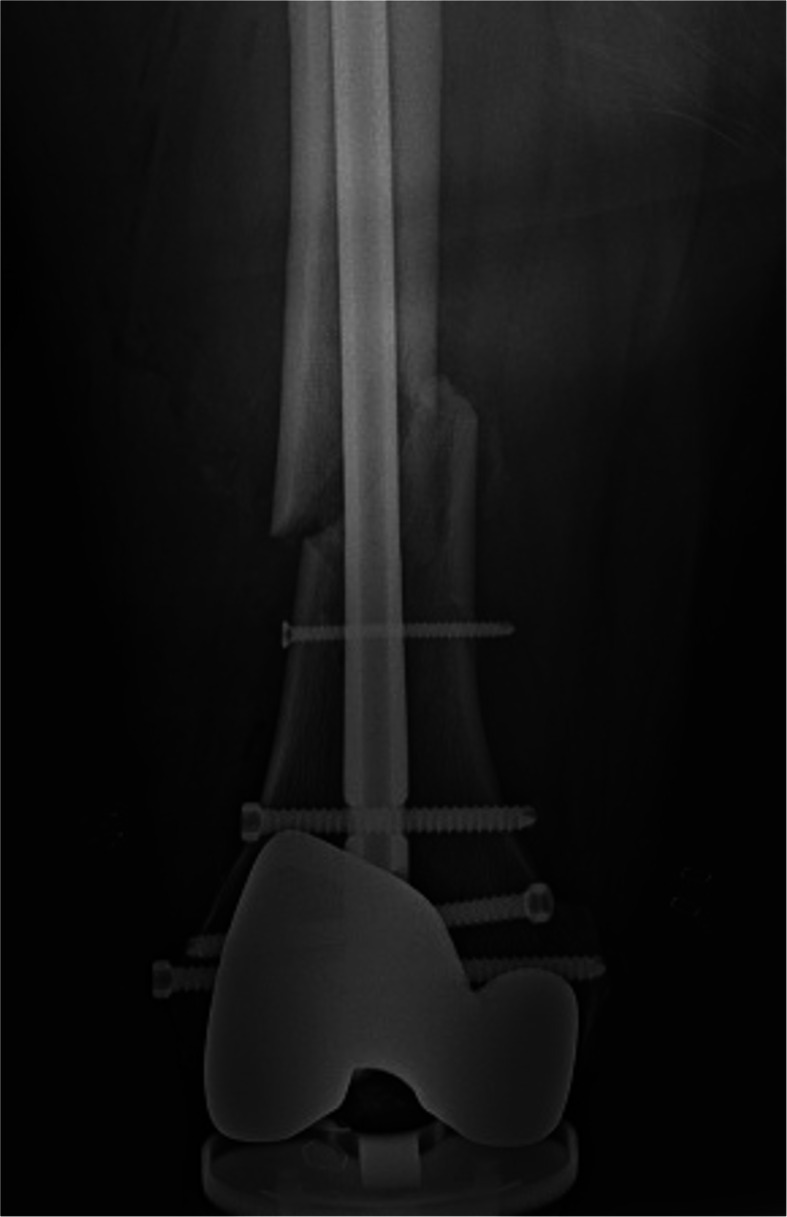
An anteorposterior X-ray shows the fracture is fixed with intramedullary femoral rodding
